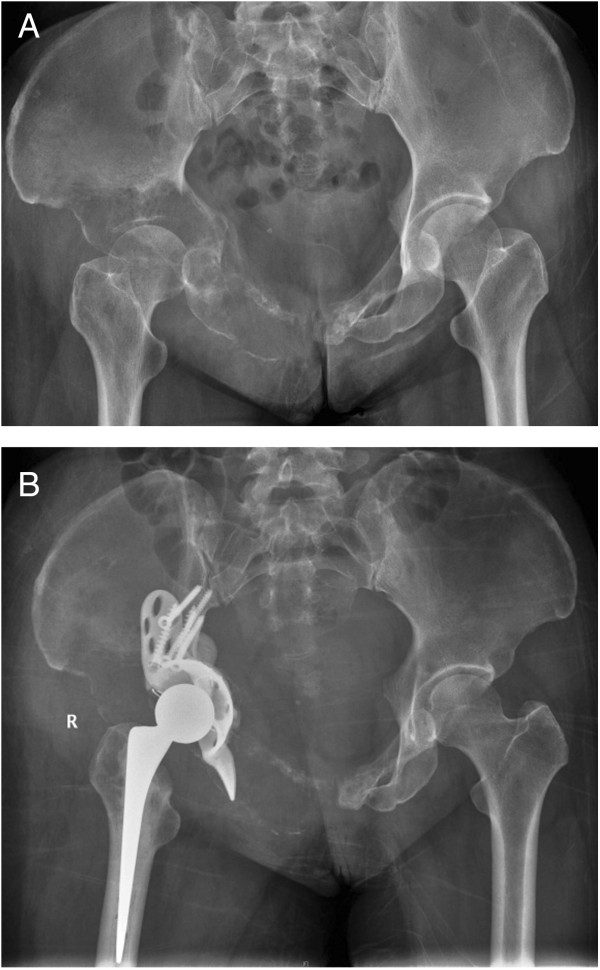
Radiograph of left hip of a 58 year-old woman with class III periacetabular metastatic disease from lung carcinoma. (A) preoperative radiograph showed a large lesion involving the superior dome and medial wall. (B) after reconstruction with anti-protusio cage, screws and total hip arthroplasty. The patient was able to walk with a cane and was still alive at 6 months after the surgery.
